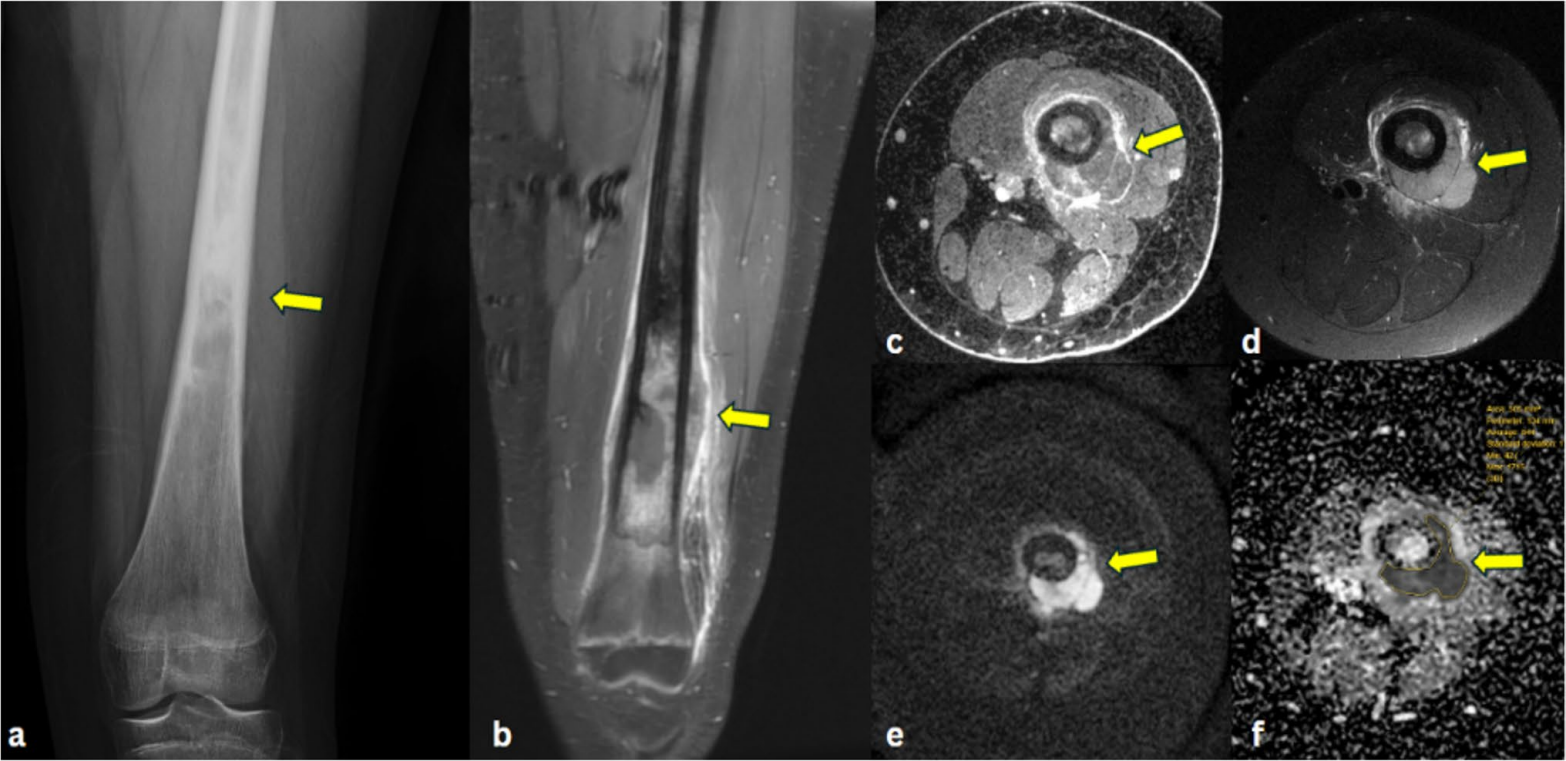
Osteoblastic osteosarcoma of the femur in a 14-year-old female. a AP radiograph of the left femur shows heterogeneous sclerosis (arrow) of the mid to distal femoral diaphysis with a wide zone of transition and aggressive periosteal reaction. b Coronal post-contrast fat-suppressed T1-weighted image shows that the lesion has an extraosseous soft tissue component (arrow). c Axial post-contrast fat-suppressed T1-weighted image shows inhomogeneous contrast enhancement of the intra- and extraosseous mass (arrow). d Axial T2-weighted fat-saturated image demonstrates relatively hypointense intramedullary tumor, consistent with sclerosis on the radiograph, and hyperintense extraosseous soft tissue mass (arrow). e Axial DW-MRI reveals intramedullary hypointense components and extraosseous hyperintense components of the lesion (arrow). f Corresponding ADC map shows hypointense signal of the extraosseous soft tissue mass (arrow), indicating restricted diffusion, with ADCmean of 0.84 × 10^−3^ mm^2^/s, ADCmin of 0.42 × 10^−3^ mm^2^/s, and ADCmax of 1.71 × 10^−3^ mm^2^/s
